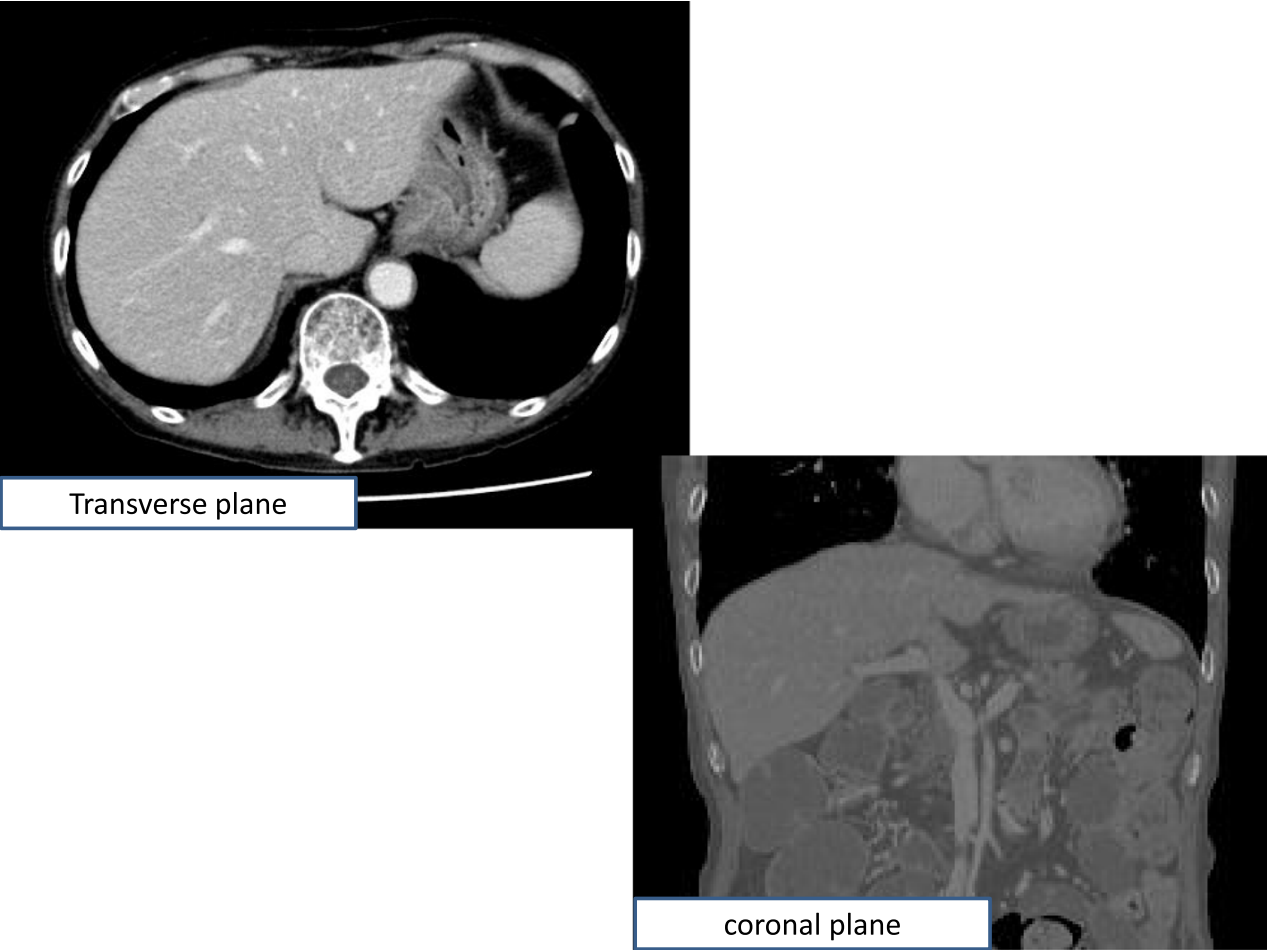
Abdominal computed tomography at the time of liver dysfunction. There were no occupational lesions in the hepatics and no dilation of either the intrahepatic or extrahepatic bile ducts
